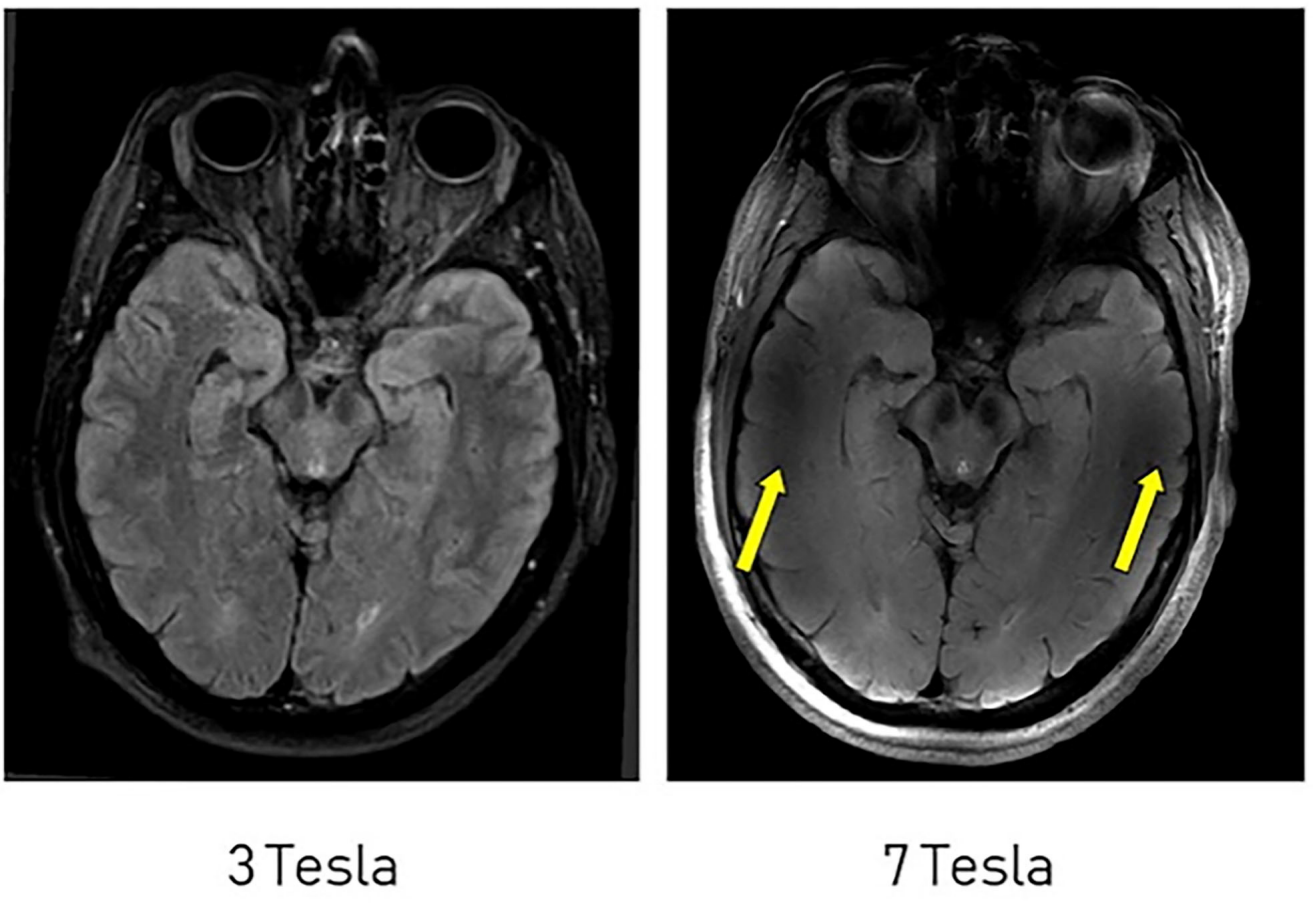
Comparison of FLAIR imaging at 3T and 7T. Artifacts are more significant near the skull base at 7T (yellow arrows). This figure is used with permission from Regnery et al. (11).
